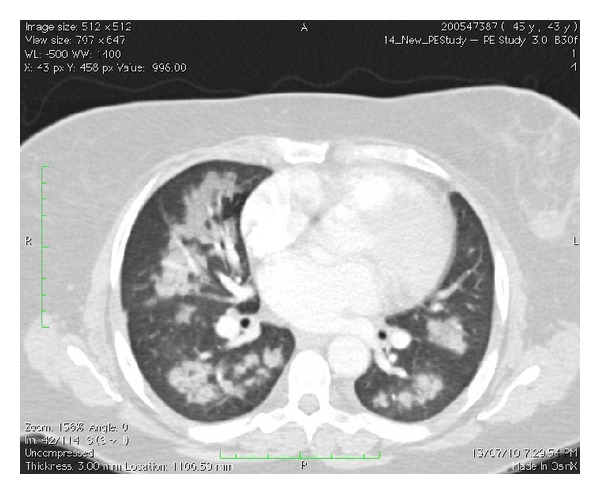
Coronal CT chest demonstrating bilateral perihilar pulmonary infiltrates consistent with diffuse alveolar haemorrhage.
